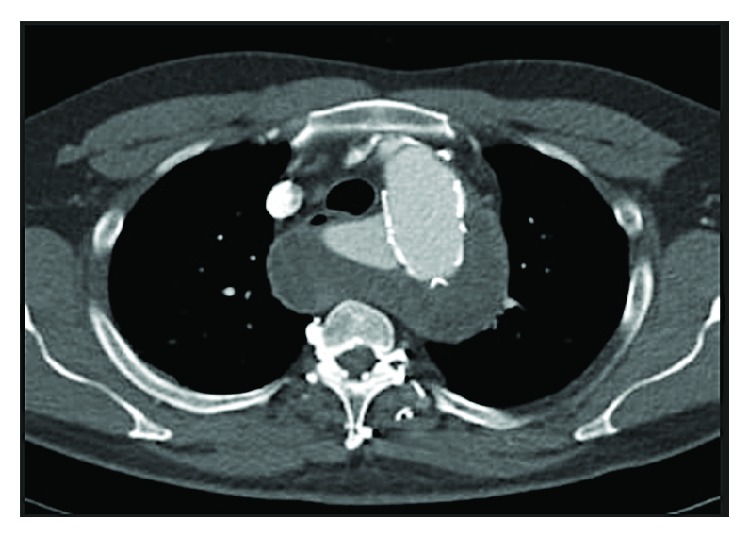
Postoperative axial CT angiography.
